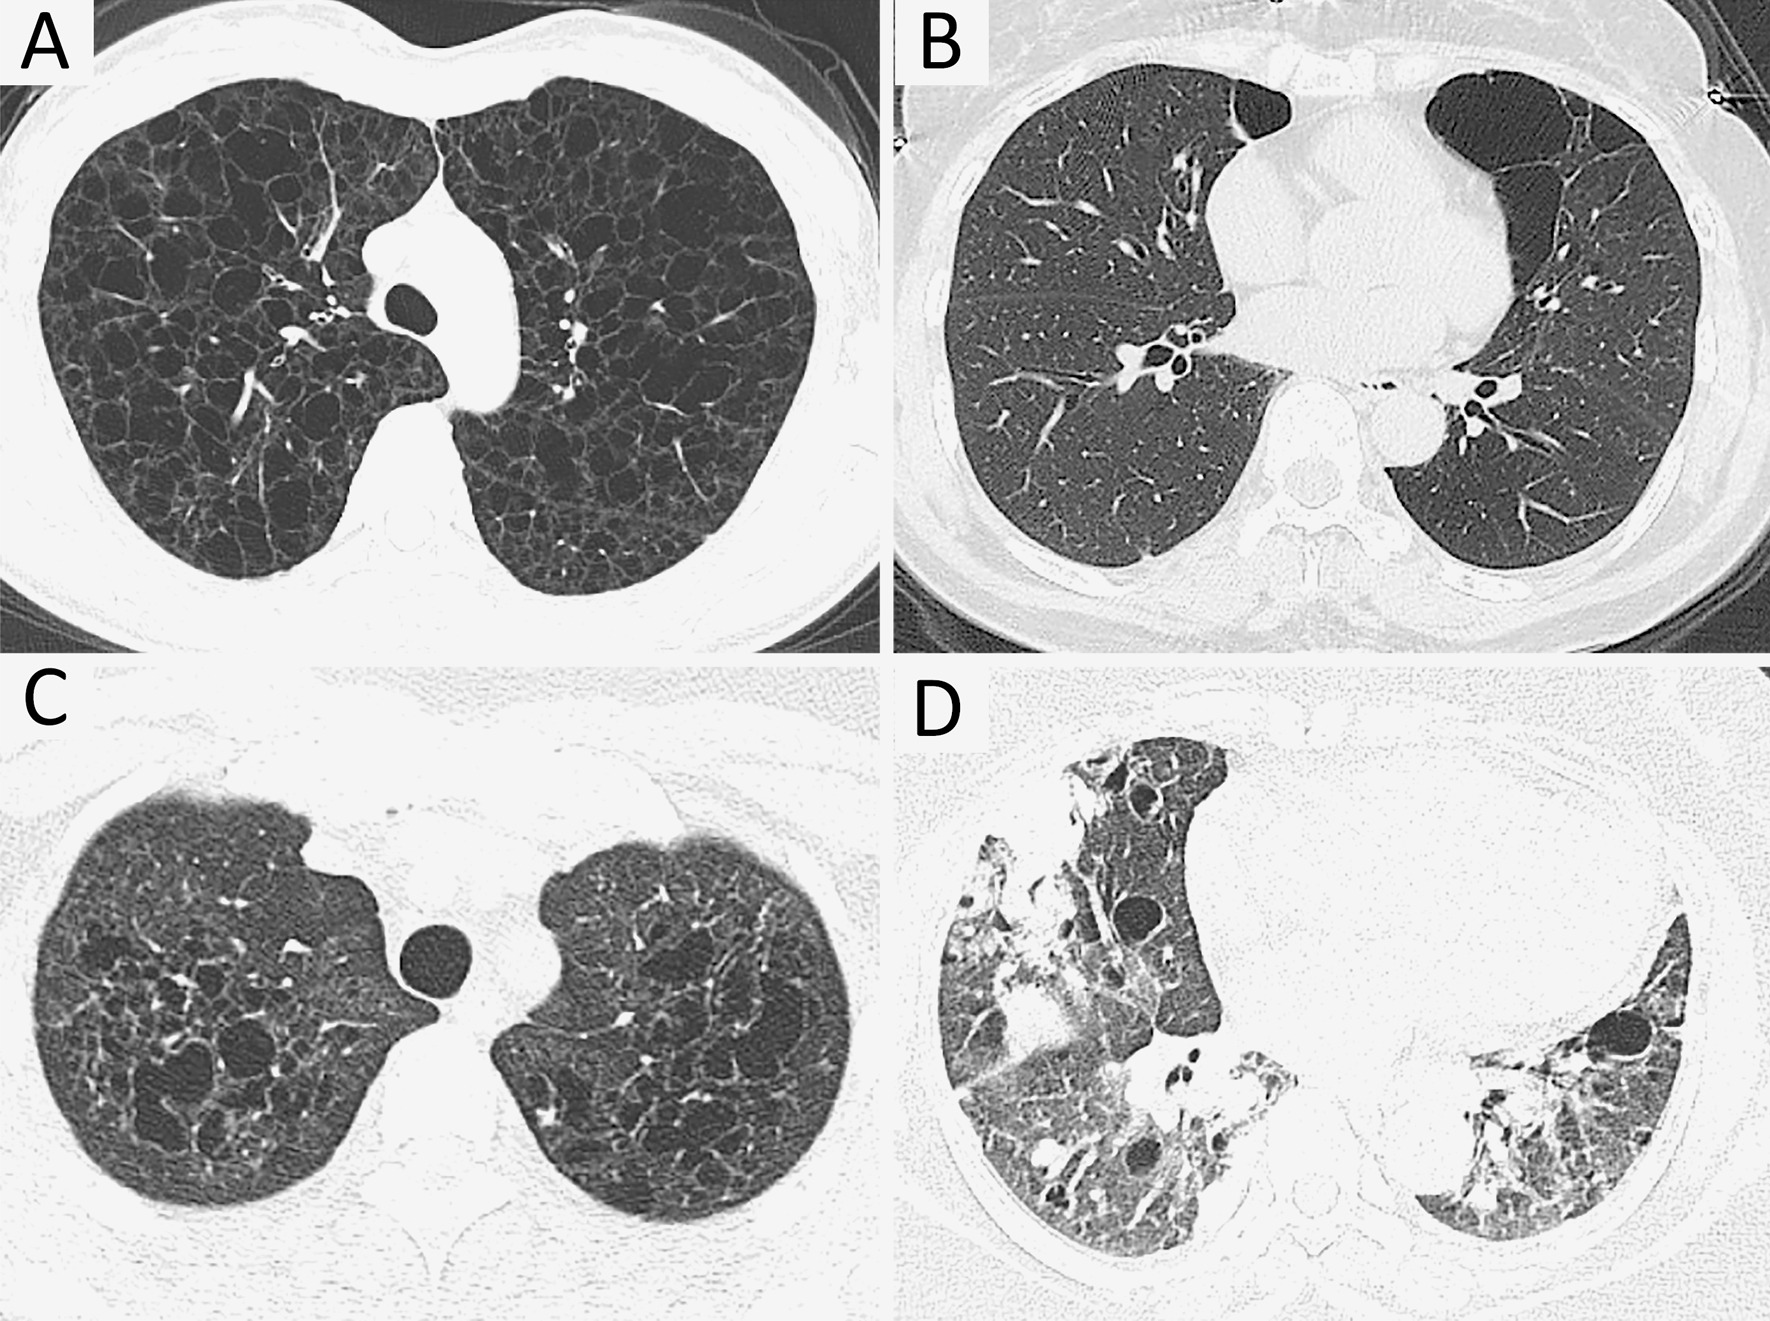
Typical images of DCLD on CT scans are suggestive of a diagnosis. A A typical lymphangioleiomyomatosis (LAM) image in a 38-year-old woman shows a evenly distributed diffuse thin-walled cysts crossover all lung fields. Pneumothorax and chylothorax are frequently seen (not shown) in patients with LAM. (2) A typical Birt–Hogg–Dubé syndrome image in a 63-year-old woman shows large and irregular cysts frequently distributed in the lower lung fields and subpleural or paramediastinal regions. (3) A typical pulmonary Langerhans cell histiocytosis (PLCH) image in a 32-year-old woman with a history of cigarette smoking shows upper and middle lung field dominant distribution with irregular cysts and multiple nodules. Costophrenic regions are likely less involved (not shown). (4) A 66-year-old women with a history of Sjogren syndrome was found diffuse cystic changes in the lung. Images on CT show randomly distributed cysts that are larger than cysts usually presented in LAM as well as patchy infiltrates and nodules. Light chain deposition disease was diagnosed after thoracoscopic lung biopsy
